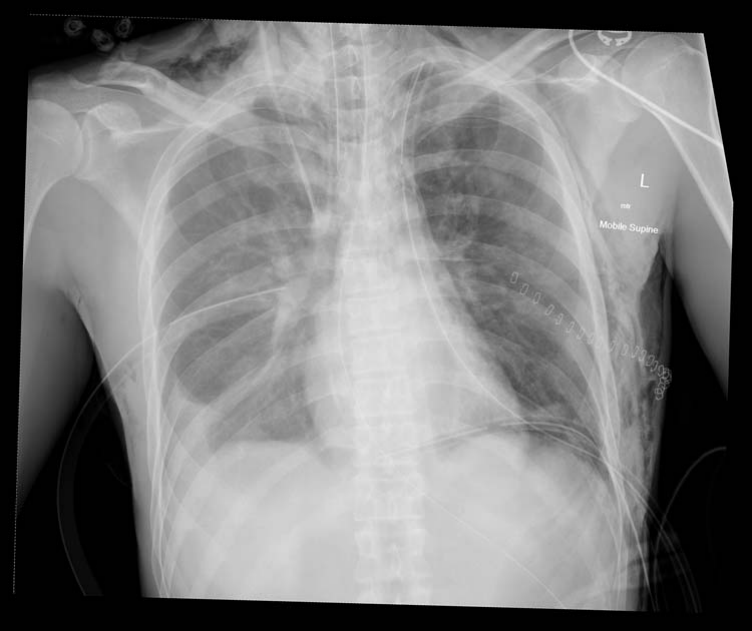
Post operative chest X-ray with bilateral chest drains and residual subcutaneous emphysema.
